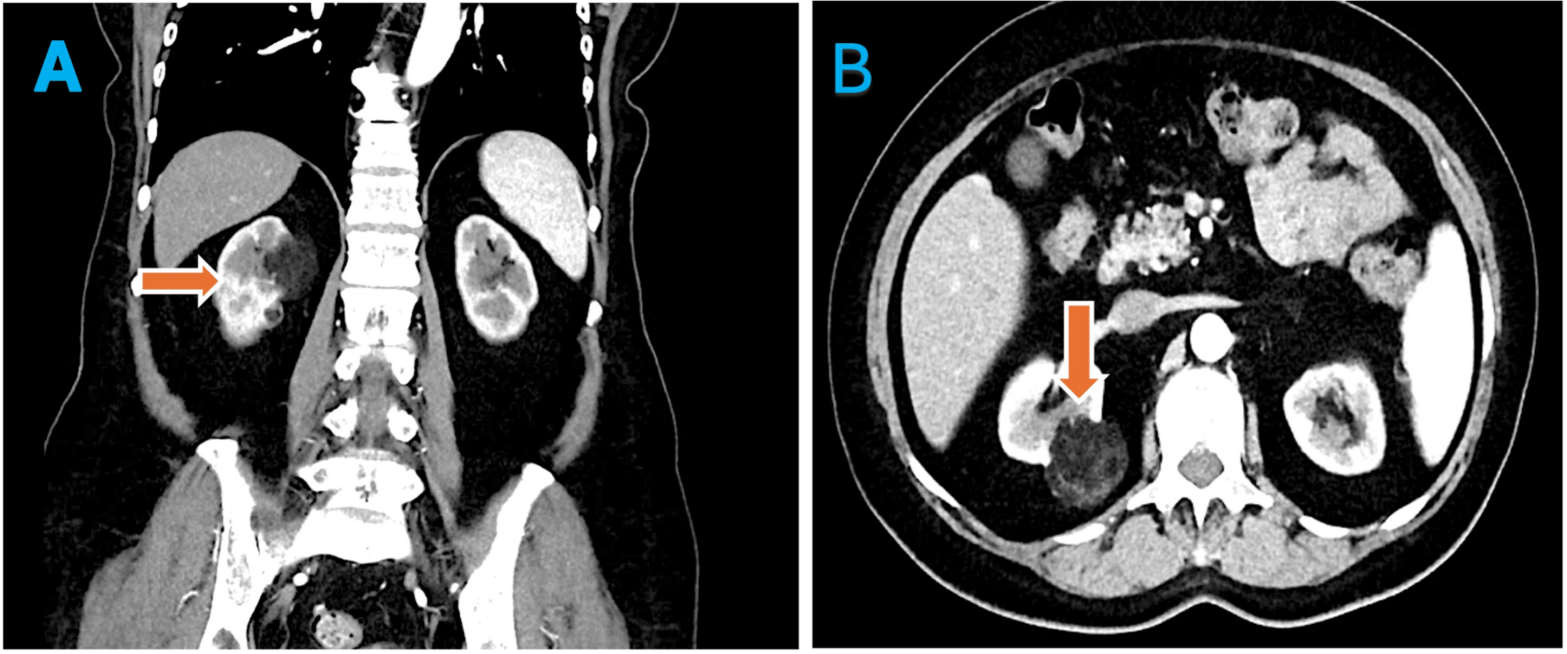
Pre-procedure CT angiogram. (A) Coronal section: Orange arrow indicates the normal-sized right kidney. (B) Axial section: Orange arrow highlights the right renal angiomyolipoma.
